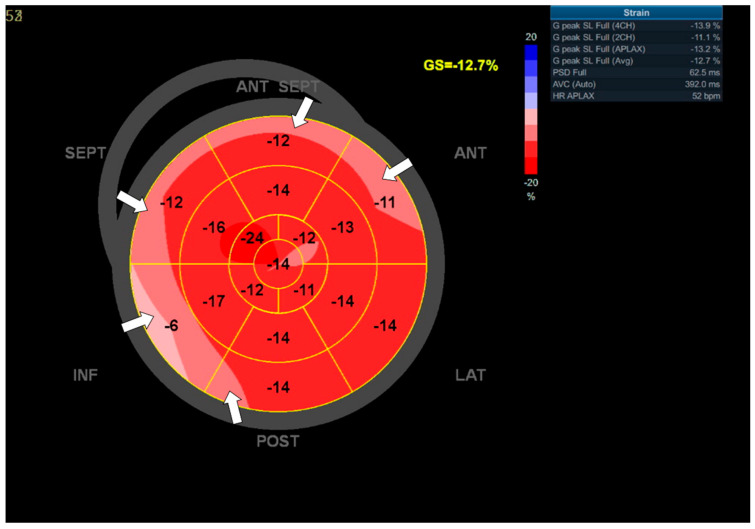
Global longitudinal strains (GLS) of the left ventricle (LV) measured by 2D speckle tracking echocardiography in Patient 1. ‘Bull’s-eye’ representation of the regional strains. Deteriorations are observed in the basal segments of the LV walls (arrows). GLS is −12.7%.
